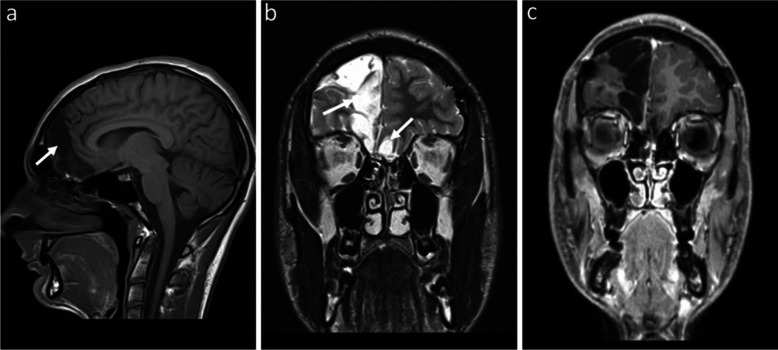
Follow–up brain magnetic resonance imaging in an 18-year-old boy, 2 years after complete tumor resection. a Sagittal T1-weighted image shows right frontal and frontobasal encephalomalacia (*arrow*). b Coronal T2-weighted image shows right frontal and bilateral frontobasal encephalomalacia (*arrows*) as sequelae of prior surgery. c Coronal gadolinium-enhanced T1-weighted image shows no abnormal enhancement. No radiological recurrence was detected
